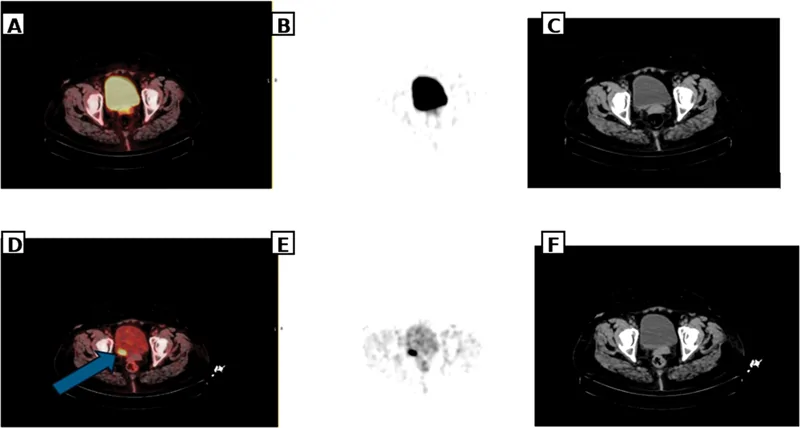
Representative case of muscle-invasive bladder cancer (BC; T2) on diuretic-enhanced dual-phase F-18 FDG PET/CT. (
